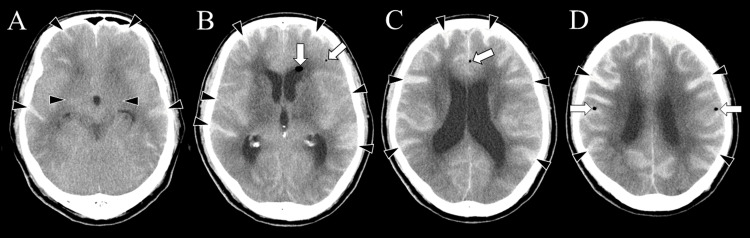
Initial non-contrast head CT at the referring hospital (Day 0, approximately 32 hours after myelography). (A–D) Axial CT images demonstrate hyperdense areas within the subarachnoid spaces along the cortical sulci (arrowheads), suggestive of subarachnoid hemorrhage. In addition, multiple low-density areas consistent with intracranial air are observed (arrows), including along the falx cerebri, within the anterior horn of the left lateral ventricle, and in the cortical subarachnoid space, suggestive of pneumocephalus.
